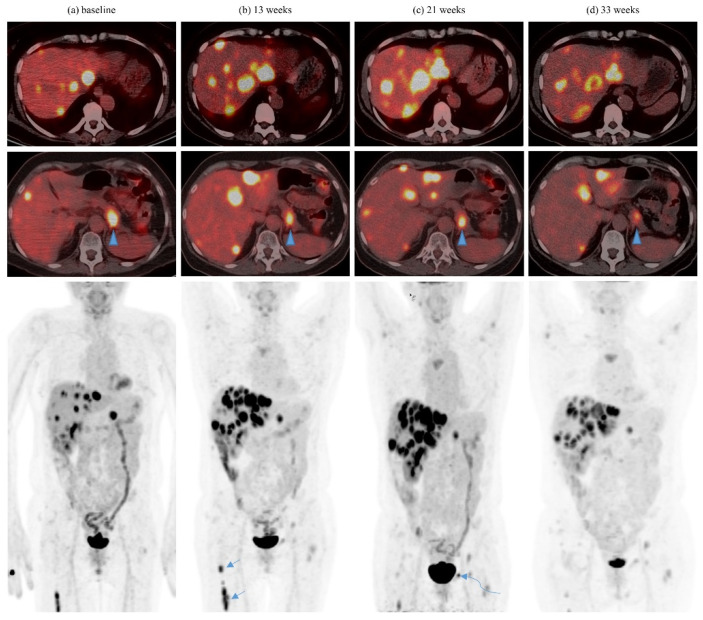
Abscopal response in a 55 year-old lady on pembrolizumab for metastatic renal cell carcinoma. (a) Baseline FDG PET/CT demonstrates multiple FDG-avid liver metastases, an FDG-avid deposit in the distal pancreas (arrowhead), and FDG-avid bone metastasis involving the right femur (arrow). (b) Assessment at 13 weeks showing dissociated response with an increase in the number, size, and FDG-avidity of liver metastases as well as an increase in the extent and FDG-avidity of the right femoral shaft metastasis but a decrease in size and FDG-avidity of the distal pancreatic metastasis. (c) Assessment at 21 weeks demonstrates the abscopal effect after single fraction radiation (8Gy) to the right femur (at 13 weeks) with a decrease in FDG-avidity of a few liver metastases. The distal pancreatic lesion shows relative stability in FDG-avidity. (d) Assessment at 32 weeks after another course of radiation (SBRT, 27Gy in three fractions) to the left pubis (at 25 weeks) reveals a more dramatic abscopal effect. There is a remarkable decrease in the size and FDG-avidity of the liver metastases and the distal pancreatic metastasis.
